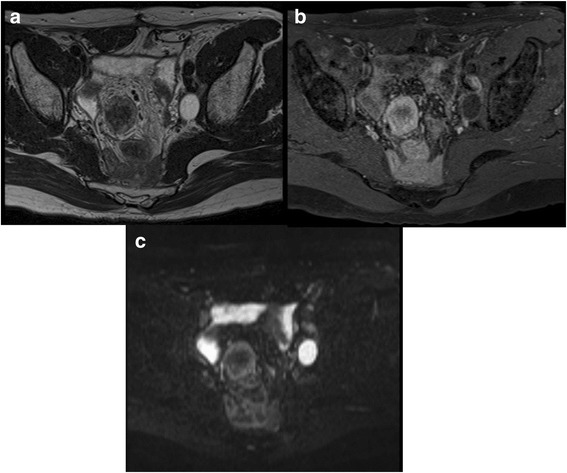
Magnetic resonance imaging (MRI) findings. a On a T2-weighted image, the central part is hypointense and the peripheral part is hyperintense. b On a gadolinium-enhanced image, the tumor is gradually enhanced. c On a diffusion-weighted image, the peripheral part is hyperintense
